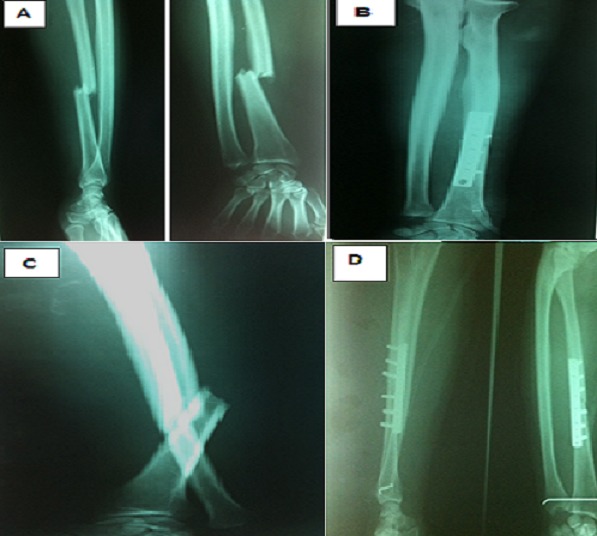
A) radiographie de face et de profil montrant une fracture de Galeazzi type 1. B) radiographie postopératoire de contrôle synthèse par plaque vissée DCP 8 trous; C) radiographie montrant une fracture de Galeazzi type 3 dorsale; D) radiographie de contrôle après synthèse par plaque vissée DCP 07 trous et embrochage de la RUD
